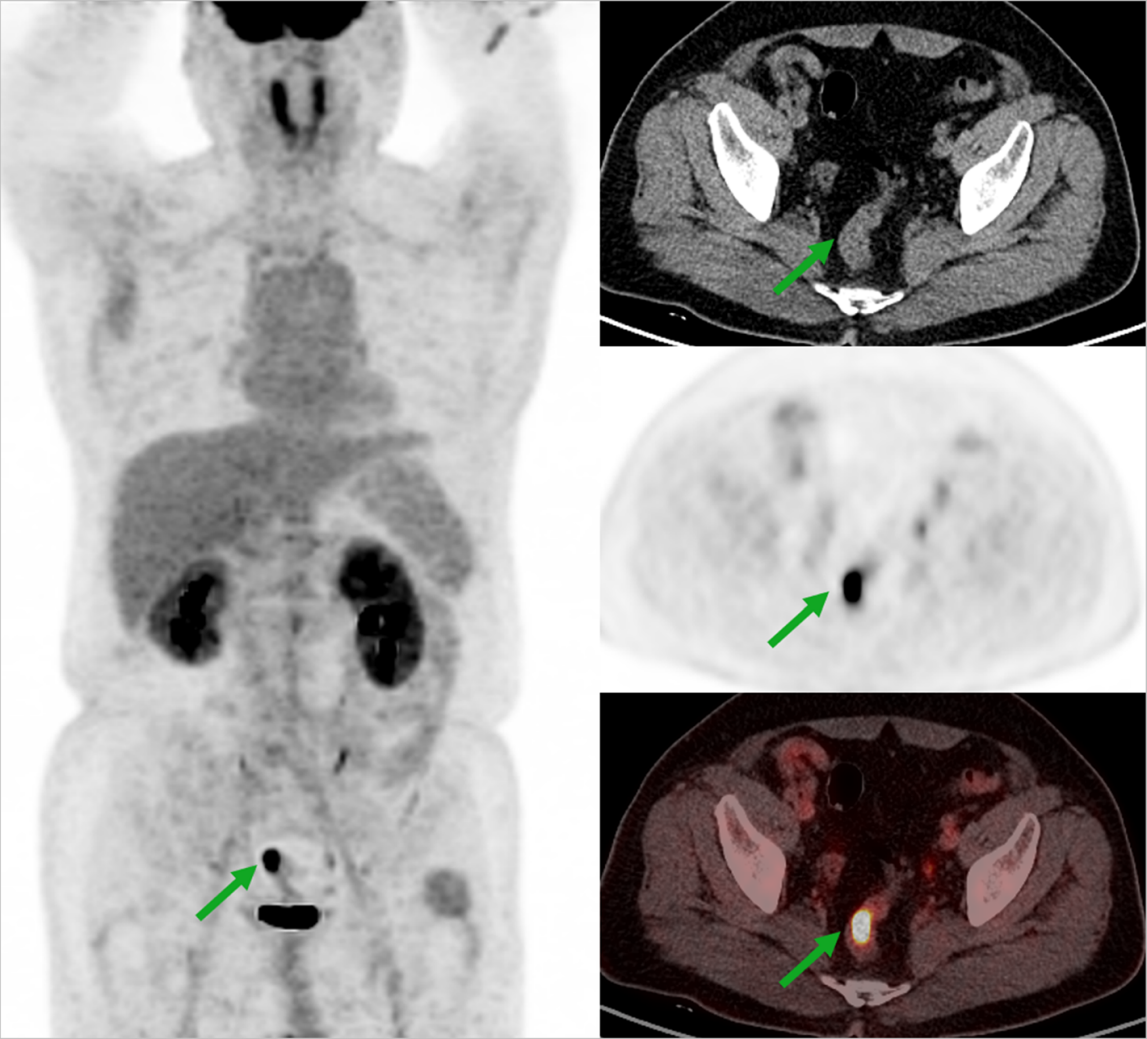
A 52-year-old man had right partial nephrectomy for papillary renal cell carcinoma. Six months after surgery, surveillance FDG PET/CT showed a focal intense uptake in the sigmoid colon (SUVmax, 14.7; red arrows), which was confirmed as benign adenoma on biopsy. There was also incidental finding of physiologic uptake by muscle in the left hip and right shoulder. The patient did not develop recurrence during the follow-up period (84 months)
